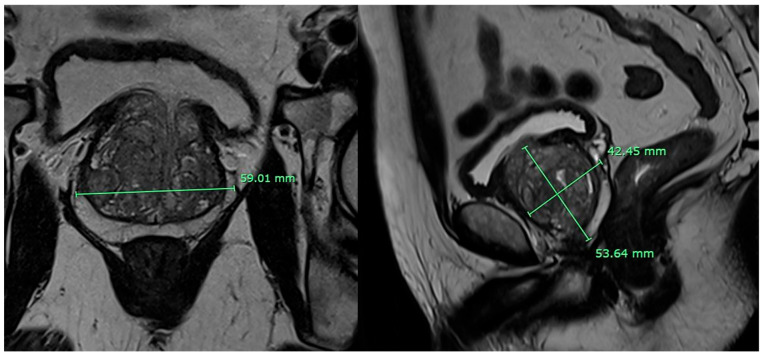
MRI images for prostate volume measurement. Axial T2-weighted image shows the maximum transverse diameter (59.01 mm). Sagittal T2-weighted image demonstrates the superoinferior (53.64 mm) and anteroposterior (42.45 mm) diameters. The calculated prostate volume is approximately 68 mL.
